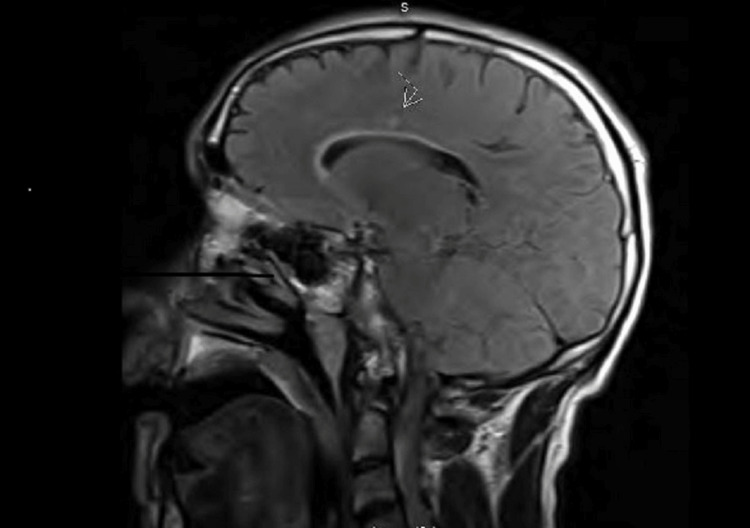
T2 FLAIR sagittal section of the brain MRI The sagittal view shows a T2/FLAIR hyperintense lesion in the periventricular white matter (arrow points to the lesion)
